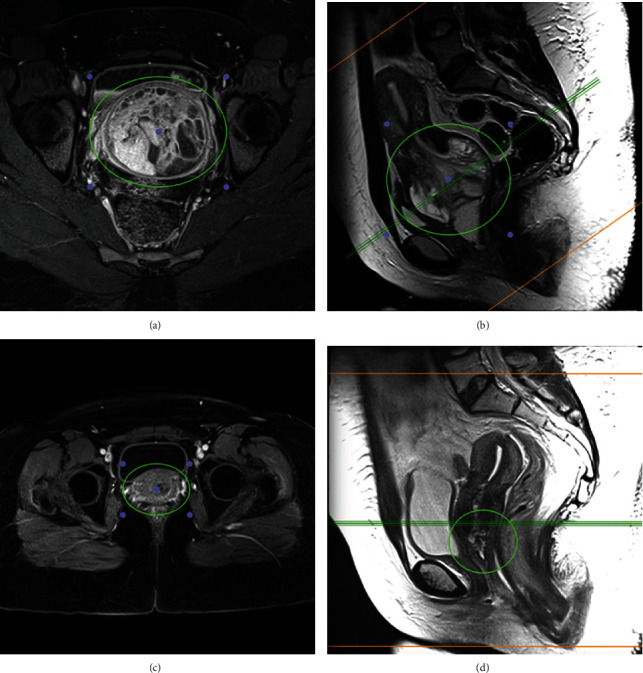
(a, b) MRI of the pelvis (axial and sagittal views) before neoadjuvant chemoradiotherapy demonstrating a heterogeneously enhanced well-circumscribed lesion measuring 3.2 cm(ap) × 6.1 cm(tr) confined within the middle compartment of the pelvis. There is association with distention of the forniceal and transitional compartments of the vagina with caudal displacement of the introitus. (c, d) MRI of the pelvis after neoadjuvant chemoradiotherapy demonstrated a significant decrease in the volume of the lesion which measured 1.3 cm(ap) × 1.2 cm(tr) remaining confined within the middle compartment of the pelvis. The residual disease is epicentred in the upper third of the vagina with cranial extension into the cervical and lower endometrial canal.
